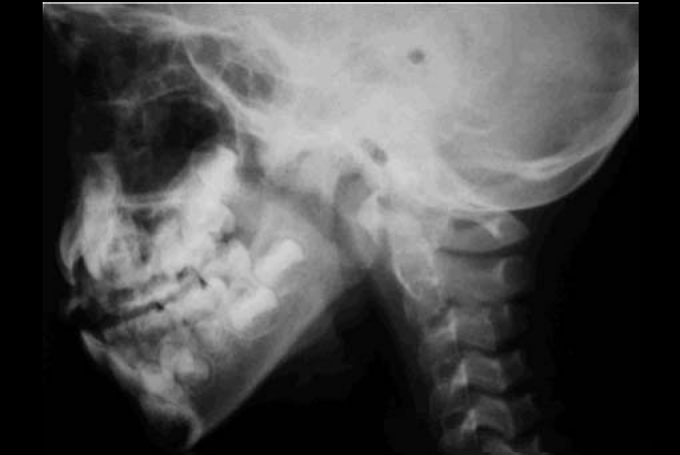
Plain x-ray paranasal sinuses profile.
